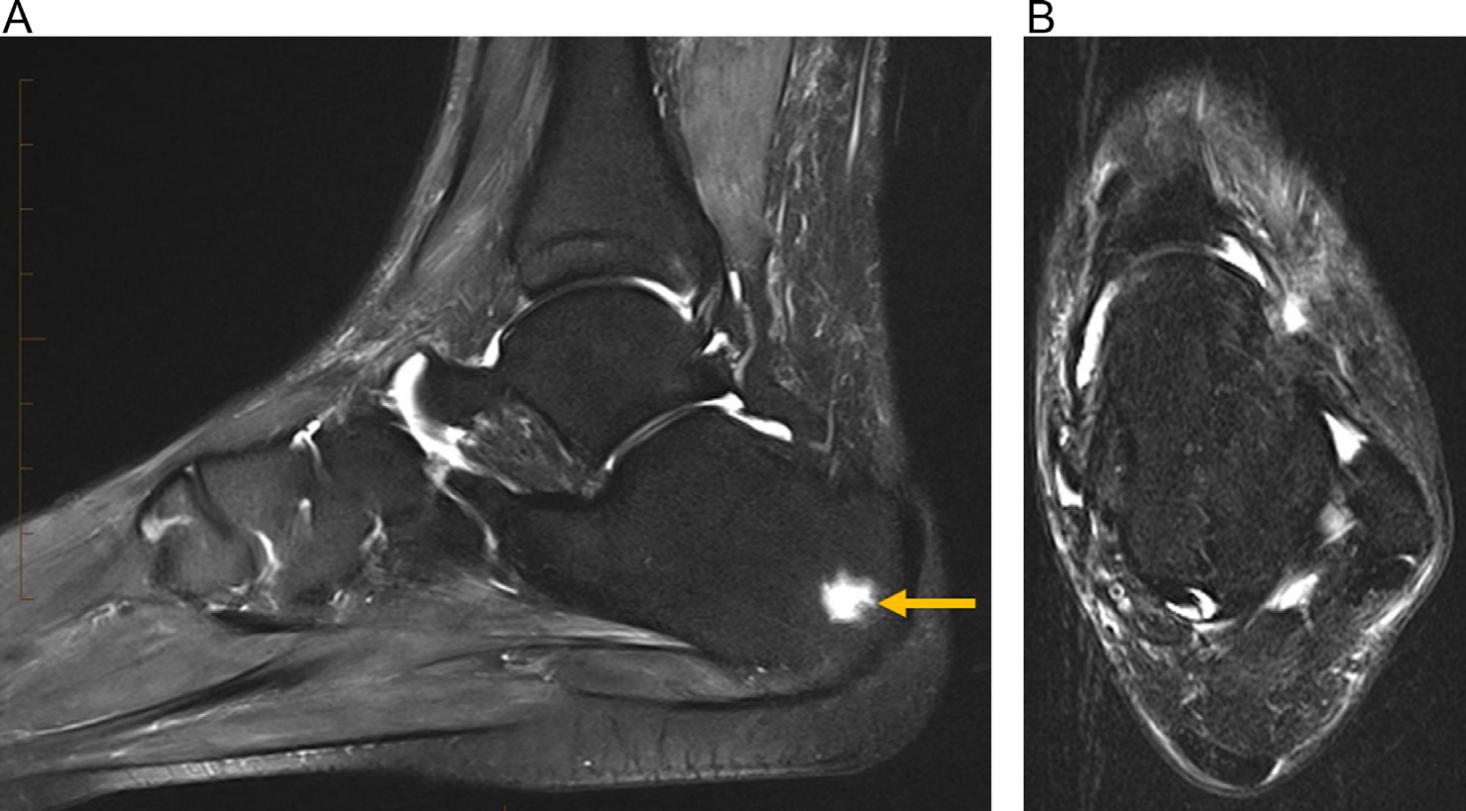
(A) Sagittal T2 fat sat image from follow up MRI of the left foot (3 months after the MRI shown in Fig. 6) demonstrates persistent focal marrow signal hyperintensity in the posterior calcaneus (arrow), although with interval resolution of surrounding marrow edema pattern and resolution of the previously noted soft tissue fluid collection. (B) Axial T2 fat sat image from follow up MRI of the left foot demonstrates complete resolution of the fluid collection that was previously seen posterior to the lateral malleolus.
